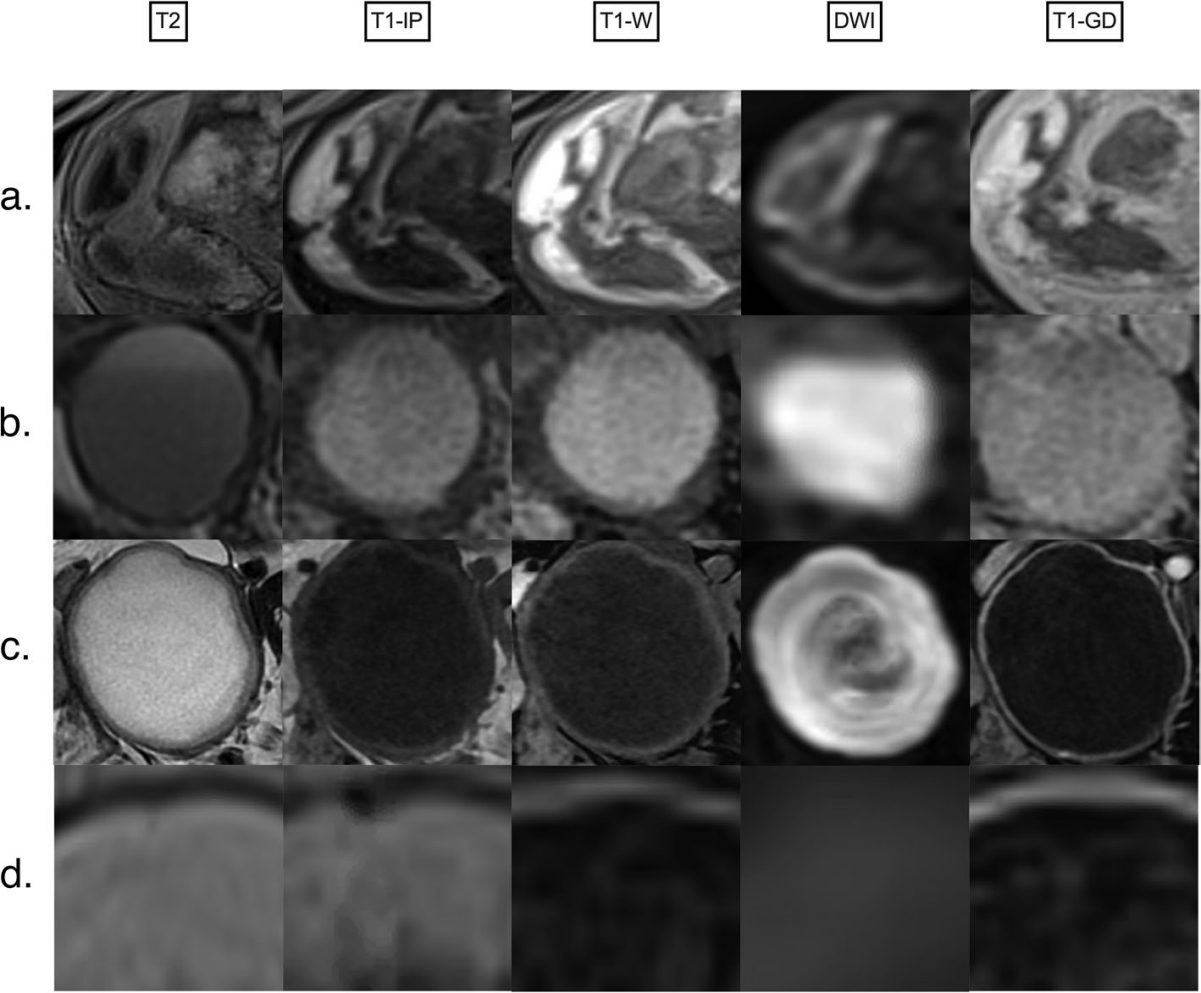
MRI assessment of non-simple fluid. First column corresponding to T2-weighted signal. Second column corresponding to T1-weighted in-phase sequence. Third column corresponding to T1-weighted water-phase sequence. Fourth column corresponding to diffusion-weighted sequence. Last column corresponding to late gadolinium T1-weighted sequence. a Hemorrhagic fluid. b Endometriotic fluid. c Proteinaceous fluid in this case: pus inside an abscess. d Lipid-containing fluid
